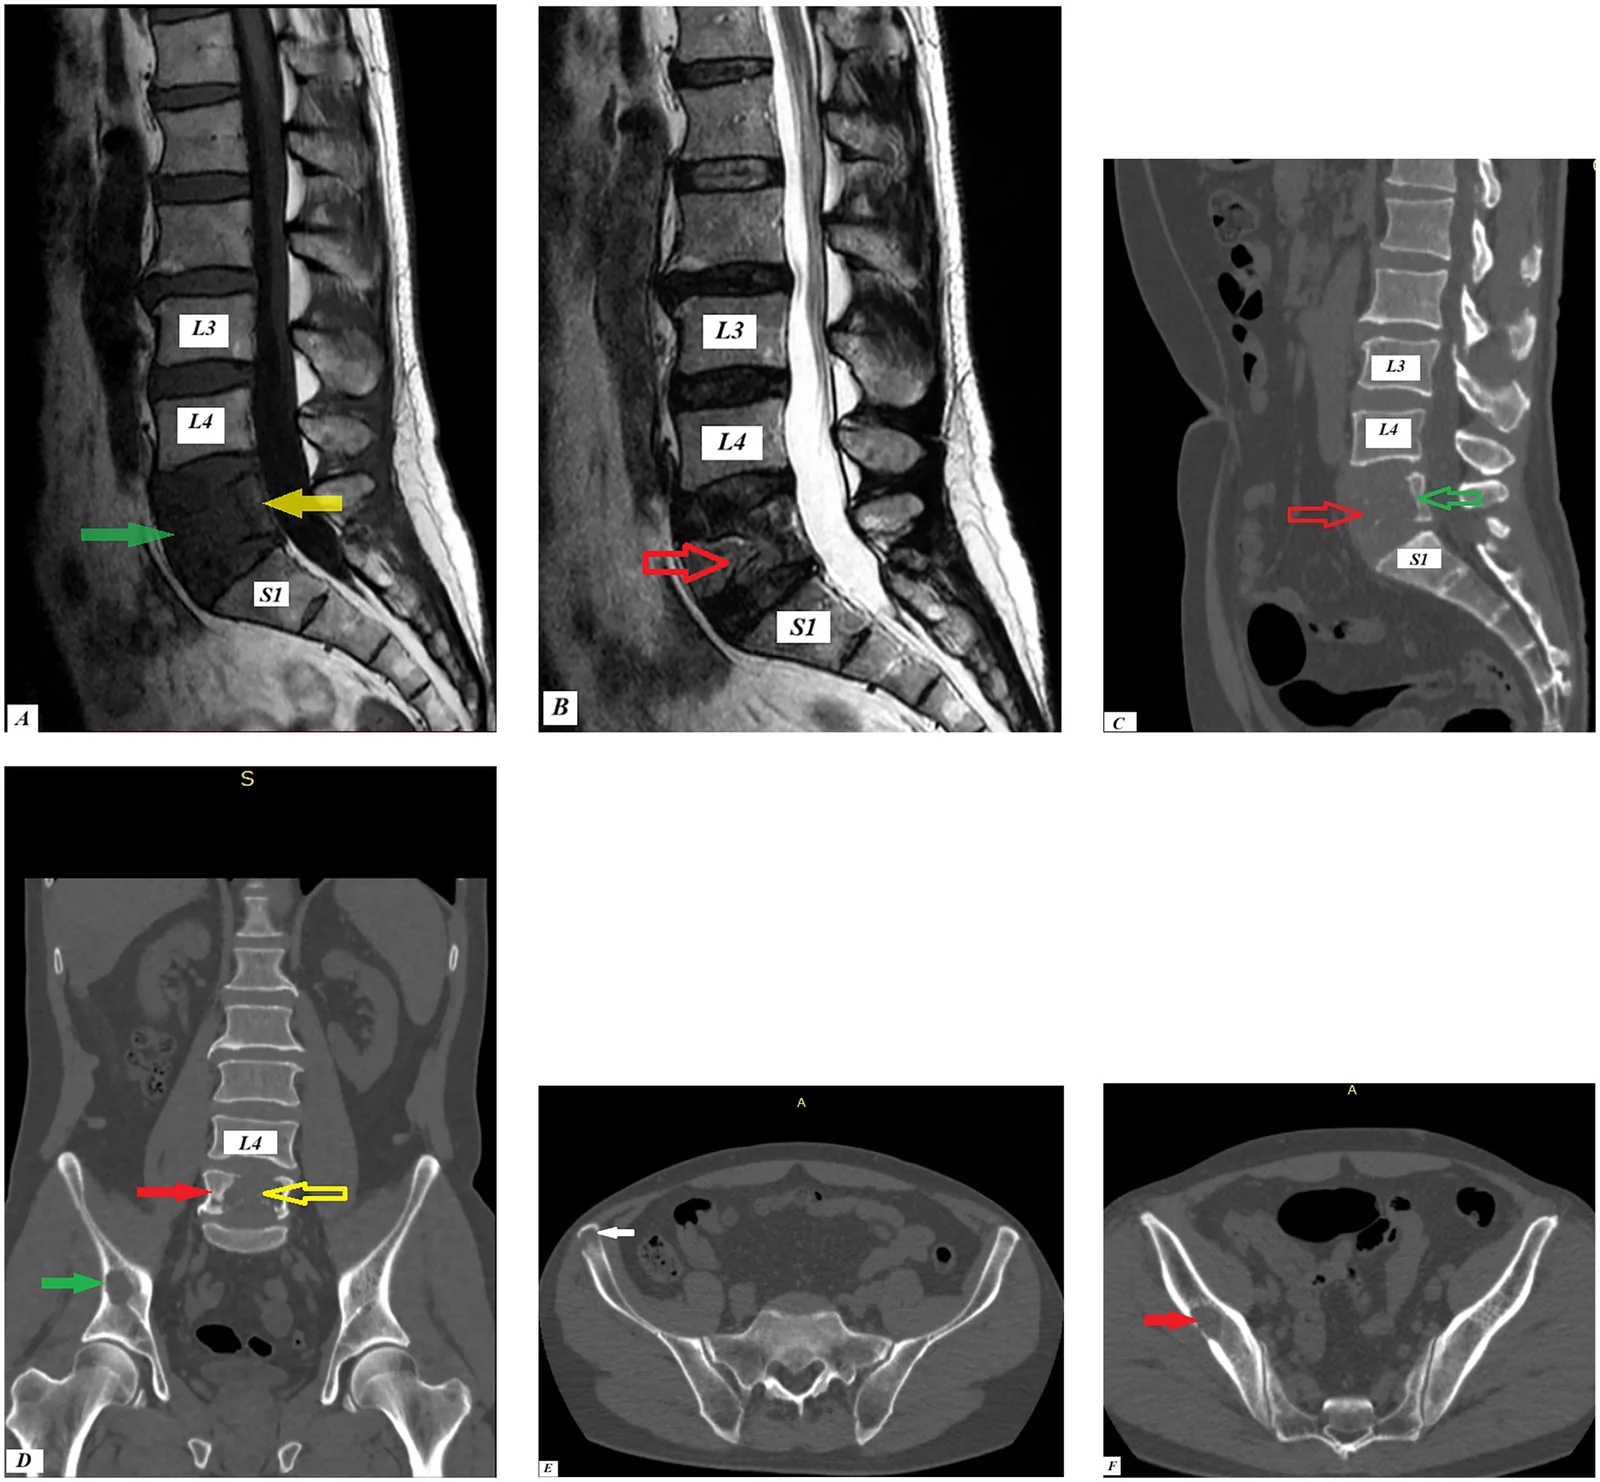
Lumbar spine MRI of the patient before surgery (A,B) and preoperative lumbar spine CT (C–F). (A) Sagittal T1-weighted image. The green arrow indicates the L5 vertebra affected by hepatocellular carcinoma metastasis. The yellow arrow shows the residual part of the L5 vertebral body. (B) Sagittal T2-weighted image. The red arrow indicates involvement of the L5 vertebra by hepatocellular carcinoma metastasis. (C) Sagittal plane. The red arrow indicates a hepatocellular carcinoma metastasis with residual bony structures. The green arrow shows the posterolateral wall of the L5 vertebral body. (D) Coronal plane. The red arrow highlights the residual portion of the L5 vertebral body. The yellow arrow indicates a hepatocellular carcinoma metastasis. The green arrow marks a destructed area of the right iliac bone (metastasis). (E) Axial plane. The white arrow demonstrates the destructed area of the right iliac wing affected by a hepatocellular carcinoma metastasis. (F) Axial plane. The red arrow indicates another destructed area of the right iliac bone infiltrated by a hepatocellular carcinoma metastasis.
